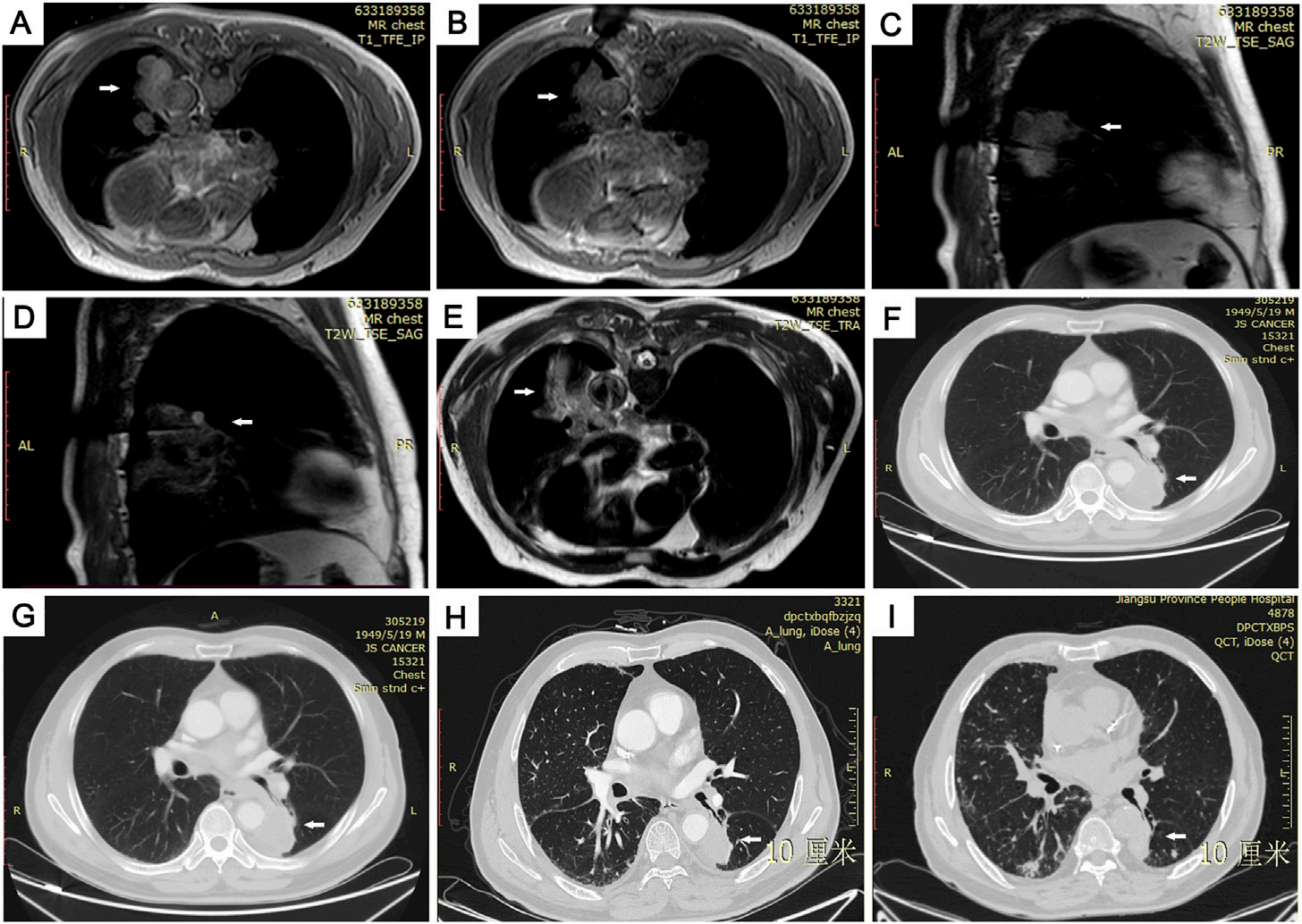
Images from 72-year-old man with 50 × 36 mm primary pulmonary adenocarcinoma in lower lobe of left lung. (A) Preoperative MRI scan and external puncture site location. (B) Ablation needle puncture reached the center of the lesion. (C,D) Due to the large lesion, a second ablation was performed by adjusting the ablation needle in real time under the sagittal image. (E) T2WI MRI scan immediately after the operation showing the surrounding ring-shaped high-signal thermal-injury response zone completely covered the ablation area with low signal and was well demarcated from the adjacent abdominal aorta. (F–I) Follow-up CT images showing the ablative zone was gradually shrinking at postoperative months 3 (F) 6 (G) 12 (H) and 16 (I).
